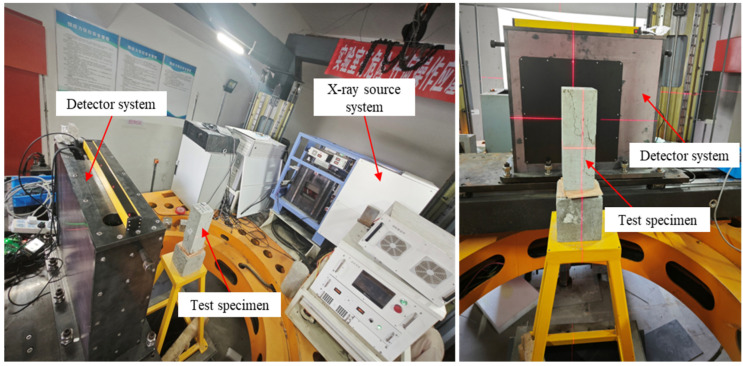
High-energy X-ray CT scanning process.
